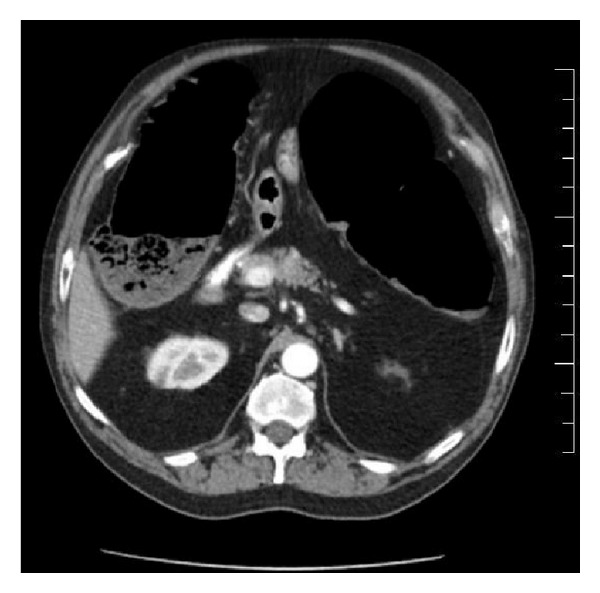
Axial CT scan of the abdomen showing the dilated large bowel.
